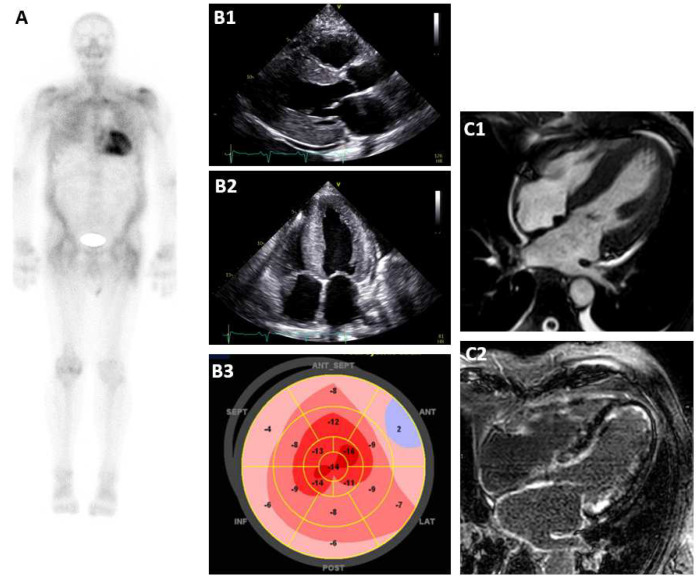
Typical findings in different imaging modalities. (A): Bone scintigraphy Perugini score 3, (B1): EC parasternal long axis, (B2): EC apical 4−chamber−view, (B3): apical sparing in strain analysis, (C1): CMR 4−chamber−view, and (C2): CMR diffuse LGE.
